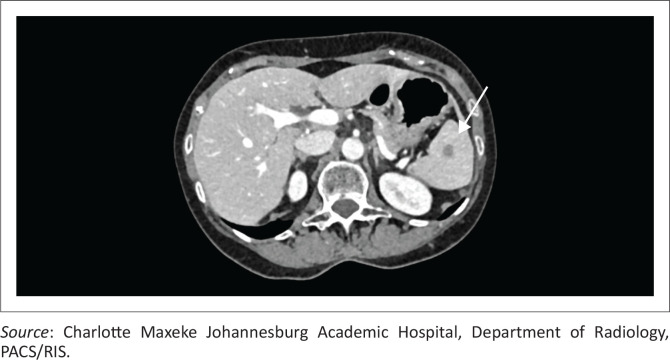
Splenic metastasis in a 44-year-old patient with primary breast carcinoma. Axial contrast-enhanced CT demonstrates a rounded hypodense lesion within the splenic parenchyma (arrow), consistent with metastatic disease.
